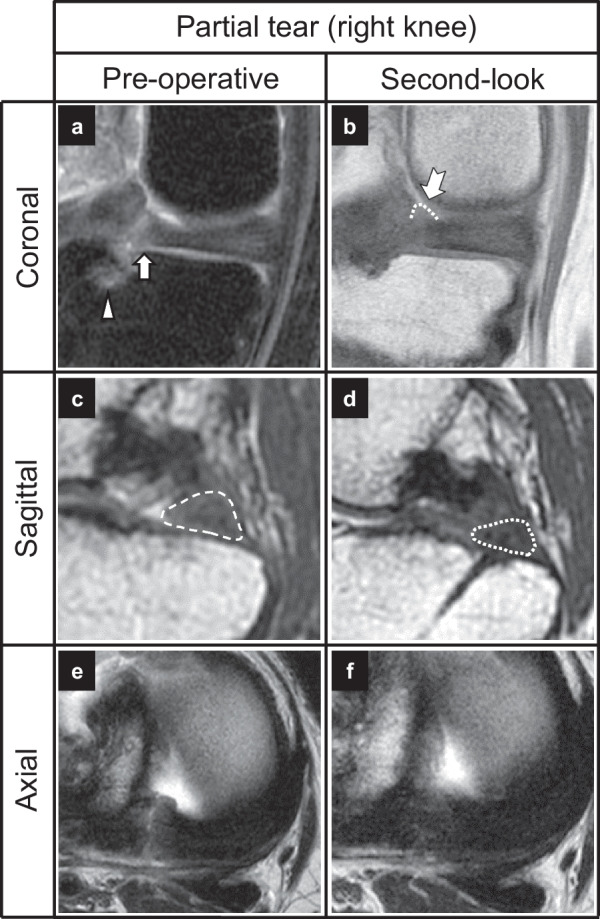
Pre- and postoperative MRI findings of a partial tear (same patient as in Fig. 3A–C). A Partial radial tear (arrow). B Suspension bridge sign (swallow-tail arrow). C Ocarina sign (dashed area). D Repaired meniscus (dashed line). E and F Axial images. *MRI* magnetic resonance imaging
